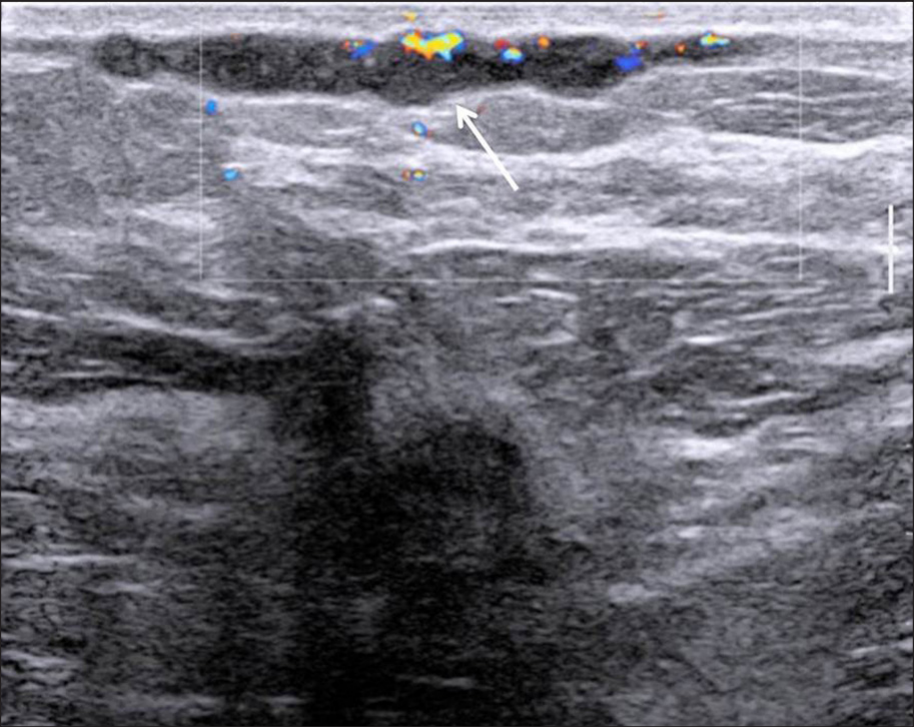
2D ultrasound with color Doppler: no vascularization inside the linear structure.
